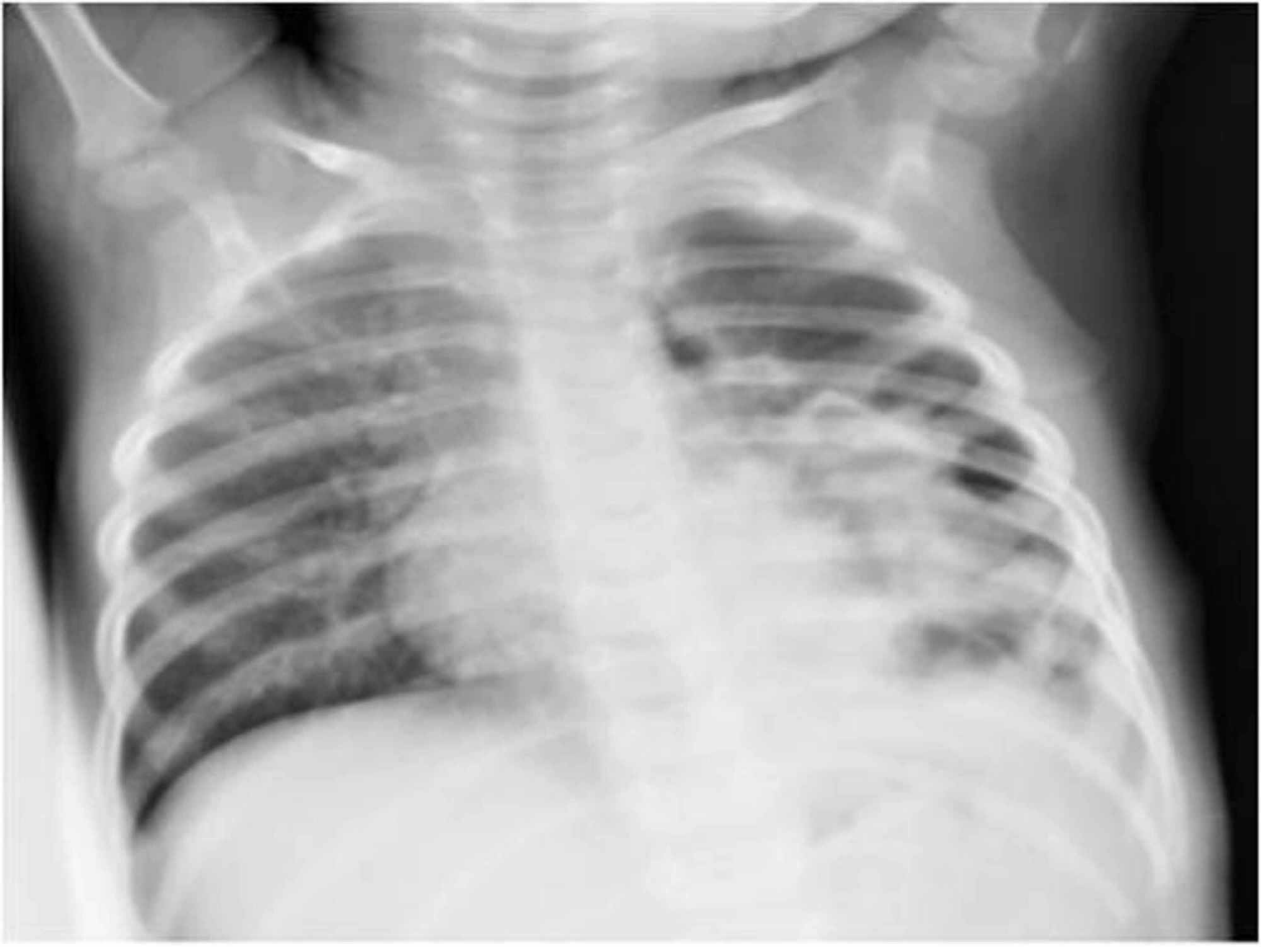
Follow-up radiograph shows the absence of intra-articular air
